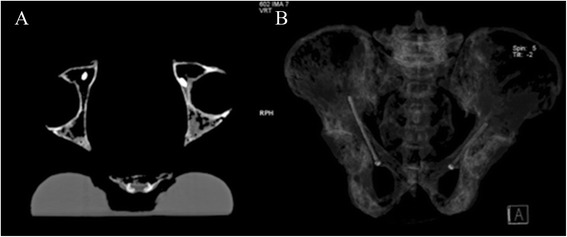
a Pelvis CT scan. b Pelvis CT 3-D reconstruction
